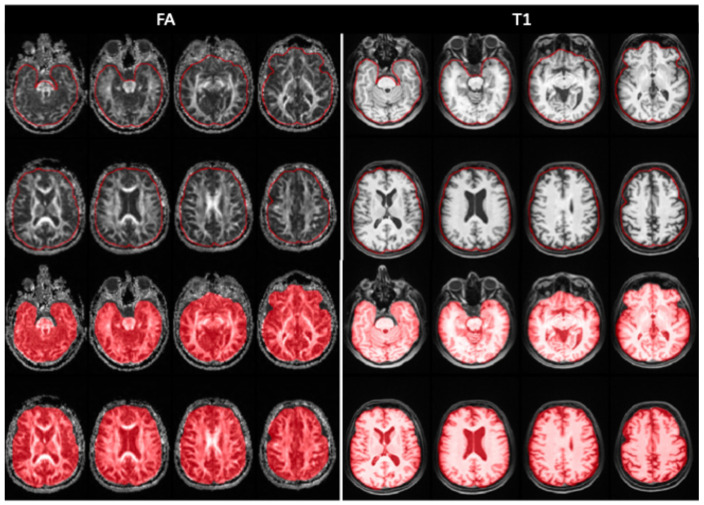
Representative examples of whole-brain segmentation on MRI fractional anisotropy (FA) images (left) and T1-weighted MPRAGE images (right). For each modality, both the segmentation contour (red outline) highlighting the boundaries of the segmented volume and the segmented mask (red overlay) for localization within the whole brain are shown.
